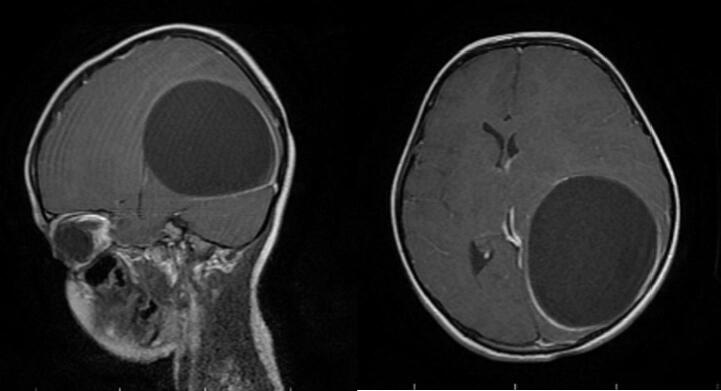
Sagittal and Axial T1W MRI with contrast showing posterior and lateral walls enhancement.
